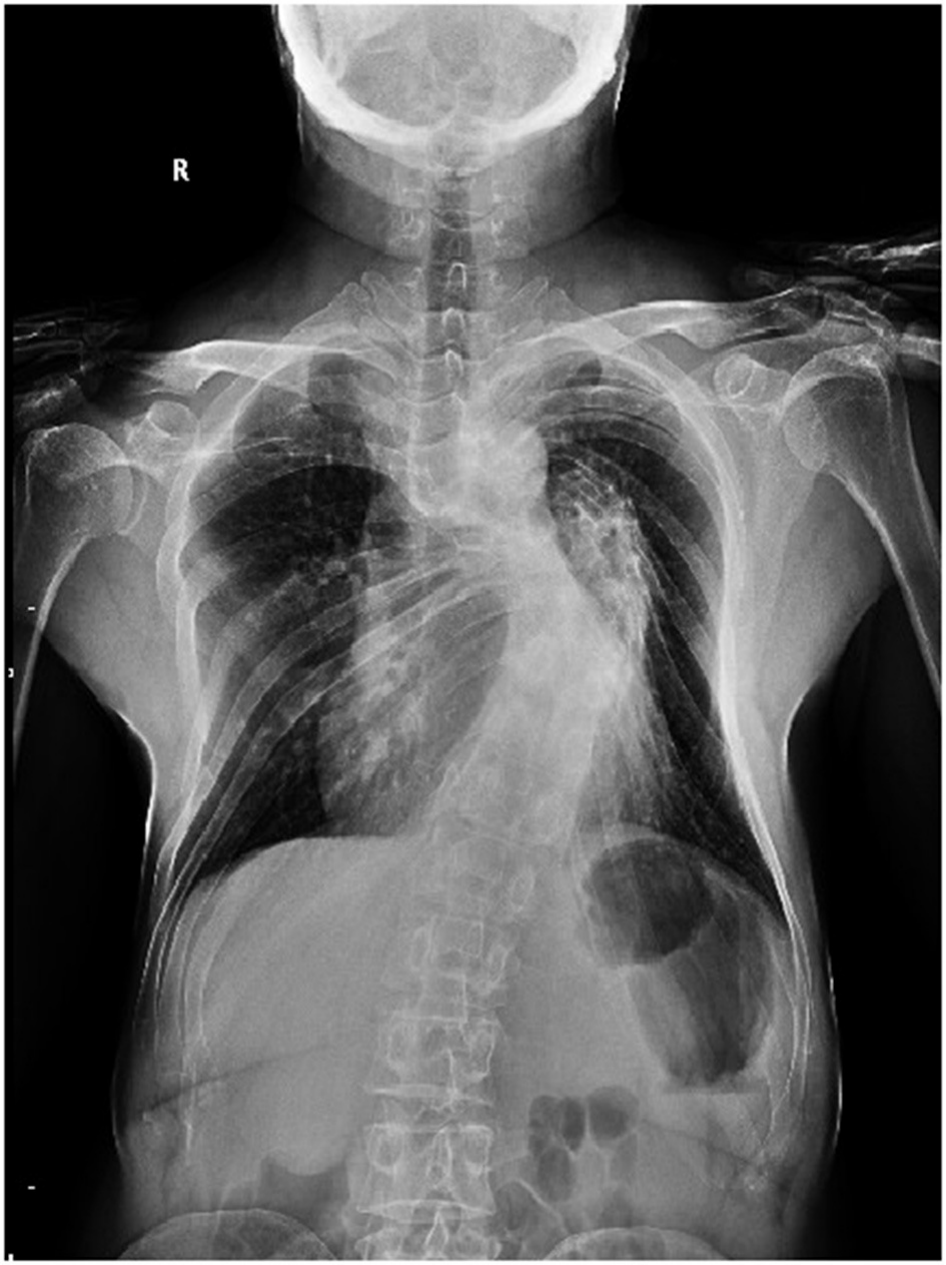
X-radiograph showing the patient with a severe thoracic scoliosis and barrel chest.
